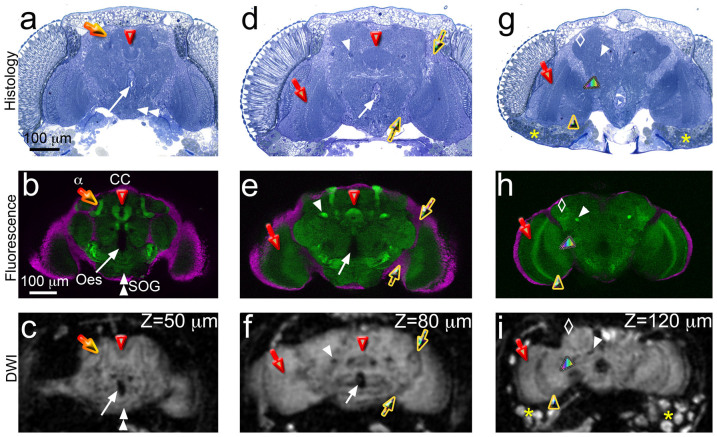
Dissection of the brain neuropil by 3D DWI. (a–c) The anterior-medial brain. (a) and (b) The most prominent structures
